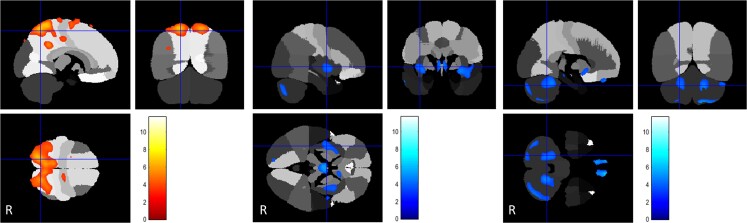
PANK2. FDG uptake in pantothenate kinase associated neurodegeneration cases (*n* = 14) compared to controls. *Left*: Areas of regional relative hypermetabolism seen in the superior parietal lobes and displayed in a yellow-scale with brighter tones indicating higher t-scores. *Middle* and *right*: Hypometabolic areas noted in the peri-insular cortex, cerebellar dentate nuclei and posterior inferior cerebellar cortex and displayed in a blue-scale with brighter tones indicating higher *t*-scores.
